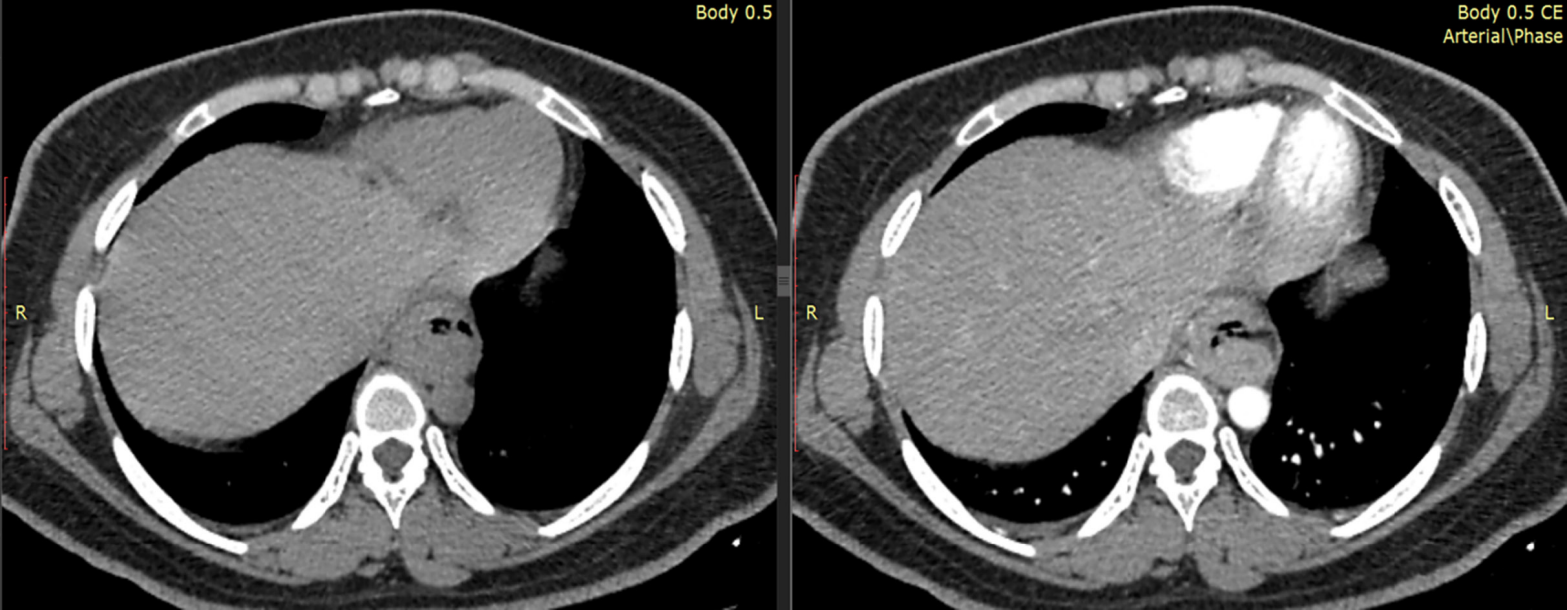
Contrast Enhanced Computed Tomography Scan (CECT‐scan) showing heterogeneously enhancing, diffuse, circumferential, asymmetric thickening of middle and lower thoracic esophagus, extending up to gastro‐esophageal junction, abutting left atrium, descending thoracic aorta and IVC anterior without luminal attenuation/defect.
